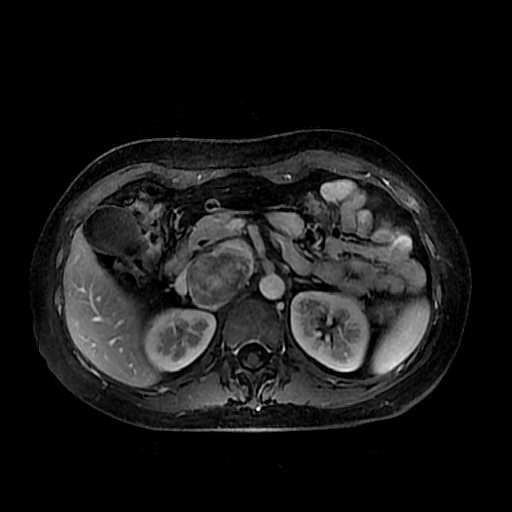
The enhancement scanning of transsected T1-weighted images showed a 6 cm heterogeneous right retroperitoneal mass, with great signal intensity on the edge and weaker in the center, compressing the inferior caval vein and the portal vein.
